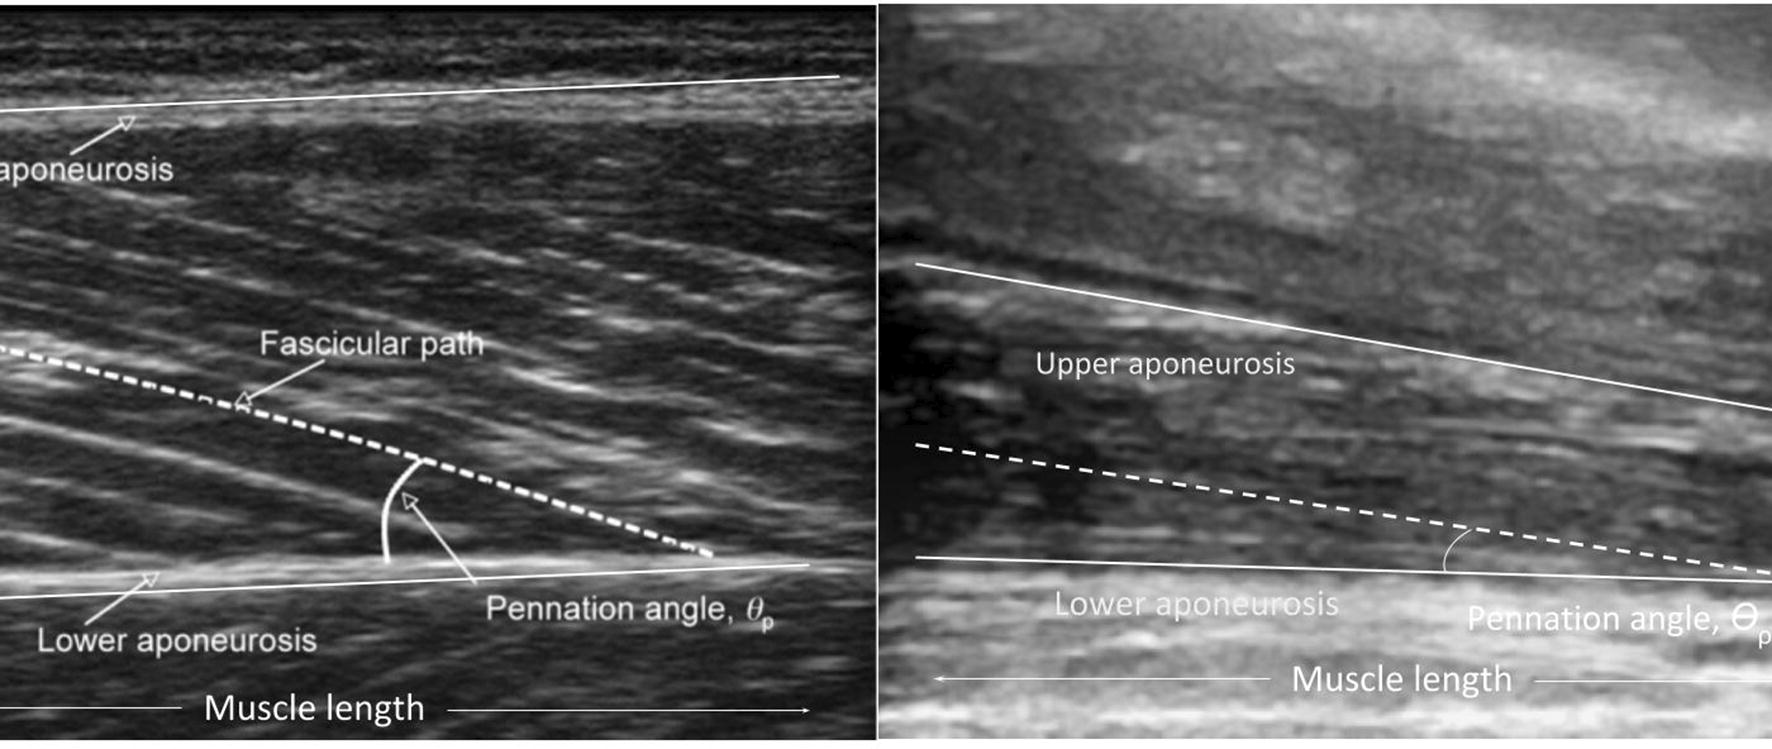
The muscle ultrasound pennation angle. The figure represents a longitudinal view of quadriceps rectus femoris muscle. The pennation angle is calculated between the intercept of fascicular path to the lower aponeurosis. Additionally, the muscle length can be measured. These two variables may be used to determine the strength of the muscle, as the lower is the angle, the lower is the length, and the lower is the strength. The right panel represents a representative reduction in pennation angle after 1 week of ICU stay
